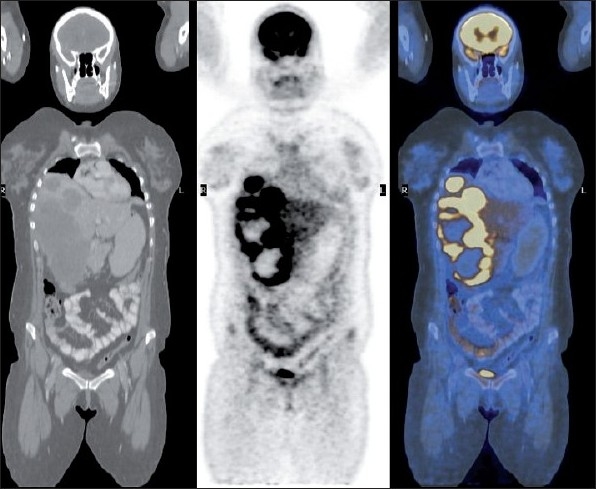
Coronal view of whole body CT, PET and fused images showing large FDG avid liver lesions with multiple necrotic areas. Celiac lymph node deposit also visualized
